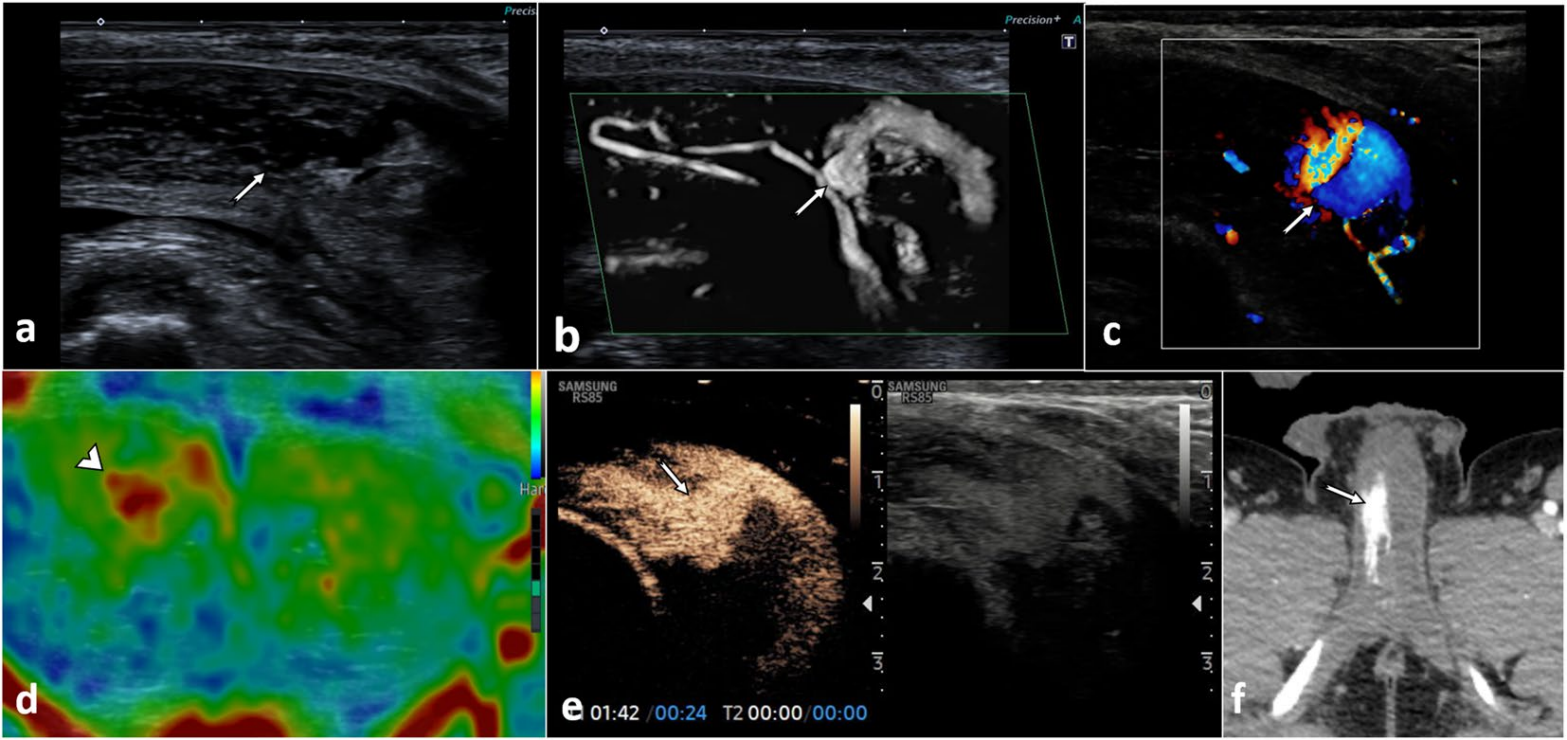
Cavernosal haematoma with an injury of the cavernosal artery and an arterio-lacunar fistula. A 26-year-old patient attended the emergency department for priapism after a perineal injury caused by a fall from a pedal bicycle. An emergent US was performed, showing in the sagittal plane in B-mode (a) and in microvascular imaging (MVI) mode (b) an abnormality of the proximal aspect of the right cavernous artery (arrow) with an outpouching, suggestive of pseudoaneurysm is demonstrated. On colour Doppler mode (c), the ‘yin-yang’ sign (arrow) is identified, representing circumferential flow within a pseudoaneurysm. In addition, elastography (d) shows a greater rigidity of the right corpus cavernosum (arrowhead), probably due to the higher blood content compared to the left corpus cavernosum. After administration of UCA (e), the injury to the cavernous artery (arrow) is demonstrated, as well as contrast extravasation and early filling of that portion of the right cavernous body, compatible with an arterio-lacunar fistula. The CT scan performed for polytrauma in the emergency department (f) shows early enhancement of the right corpus cavernosum compared to the left, as an indirect sign of an arterio-lacunar fistula
